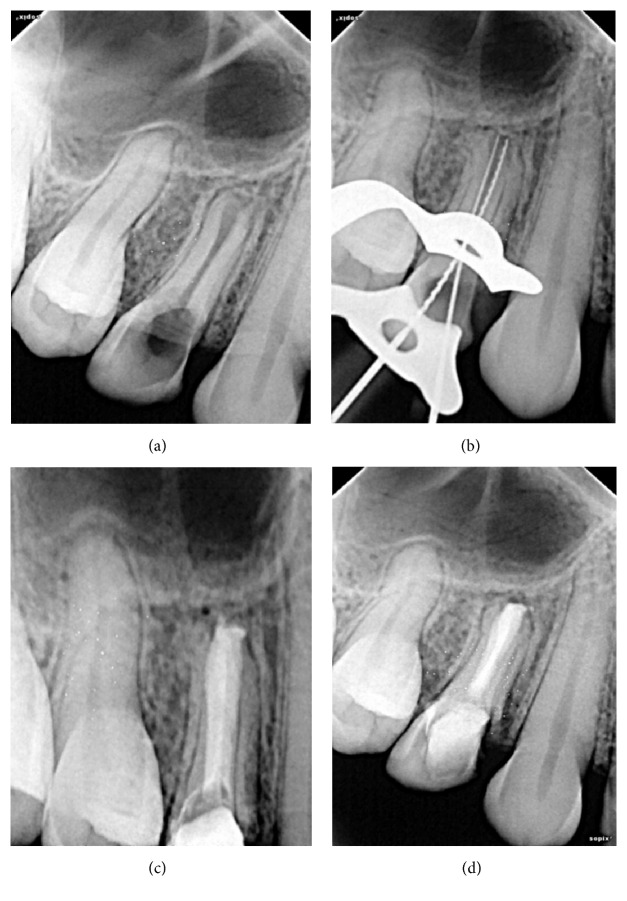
(a) Preoperative radiograph (Case  1). (b) Working length radiograph (Case  1). (c) MTA obturation (Case  1). (d) One-year follow-up radiograph (Case  1).
